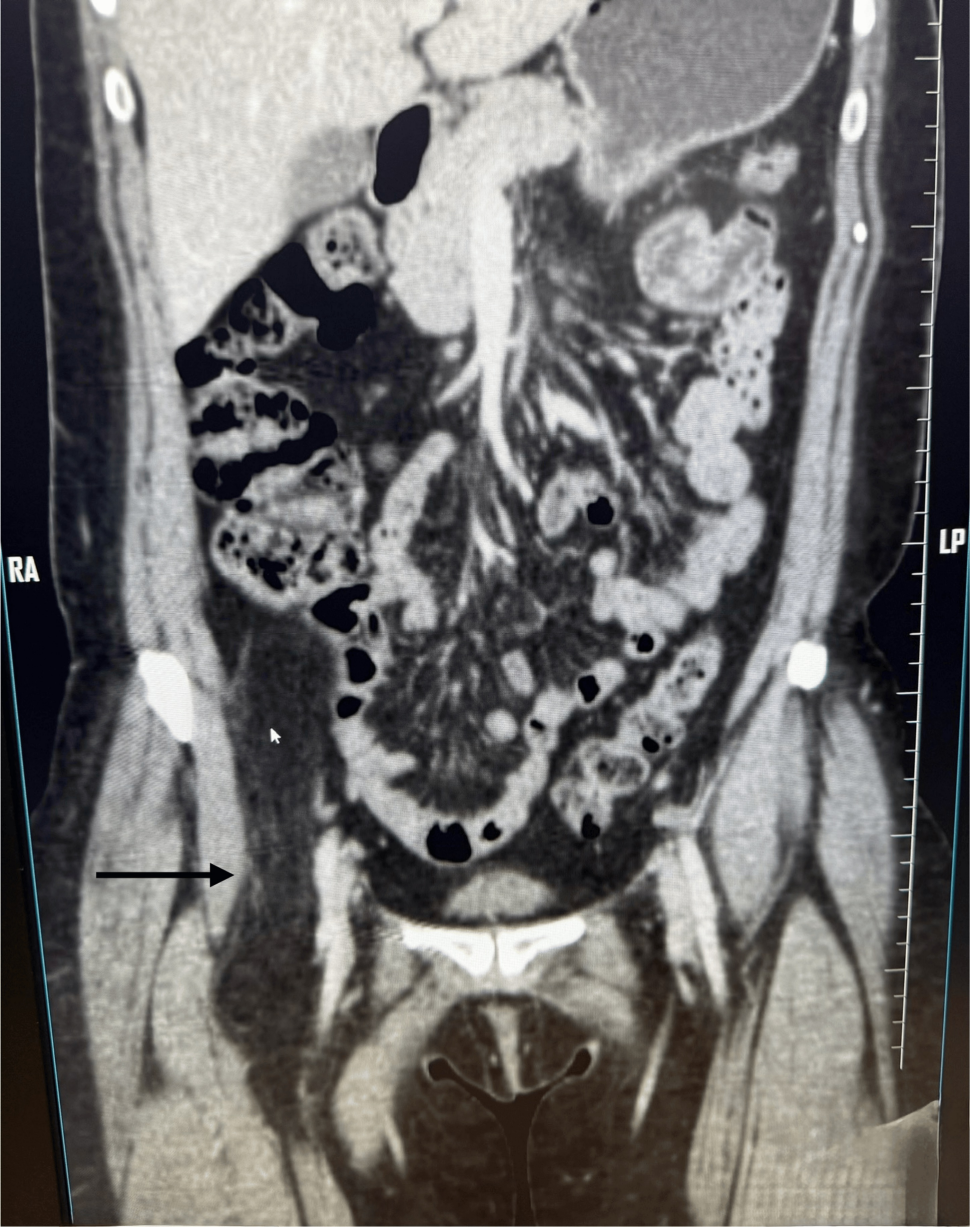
CECT scan of the abdomen showing a retroperitoneal lipoma (black arrow) extending through the femoral canal into the thigh CECT: contrast-enhanced computed tomography.
